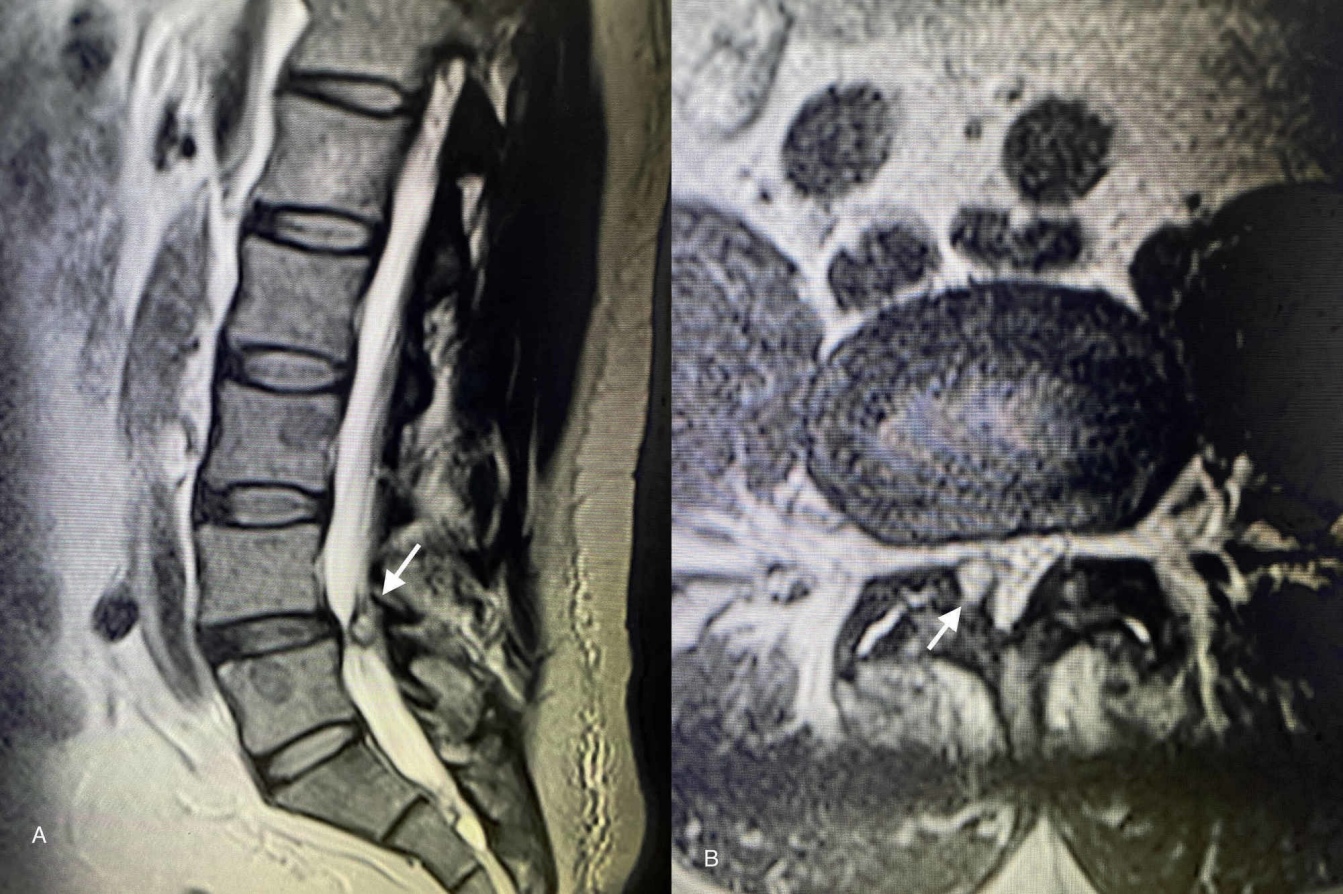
Magnetic resonance images of facet joint cysts (FJCs) at the L4-L5 vertebral level (A) Sagittal T2-weighted image demonstrating prominent FJC (white arrow) and grade 1 spondylolisthesis. (B) Axial T2-weighted image demonstrating the cyst (white arrow) arising from the right facet joint and obstructing the right lateral recess with compression of the thecal sac.
